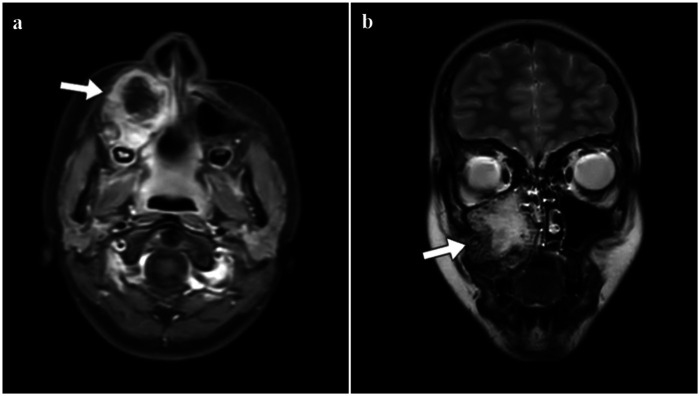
MRI of the face demonstrating a destructive maxillary lesion with soft tissue extension. (a) Axial T1-weighted contrast-enhanced MRI shows a heterogeneously enhancing mass in the right maxillary sinus (arrow), associated with erosion of the surrounding bony walls and invasion into adjacent soft tissues. (b) Coronal T1-weighted contrast-enhanced MRI demonstrates the extent of the lesion (arrow), further highlighting the destructive changes and soft tissue involvement.
